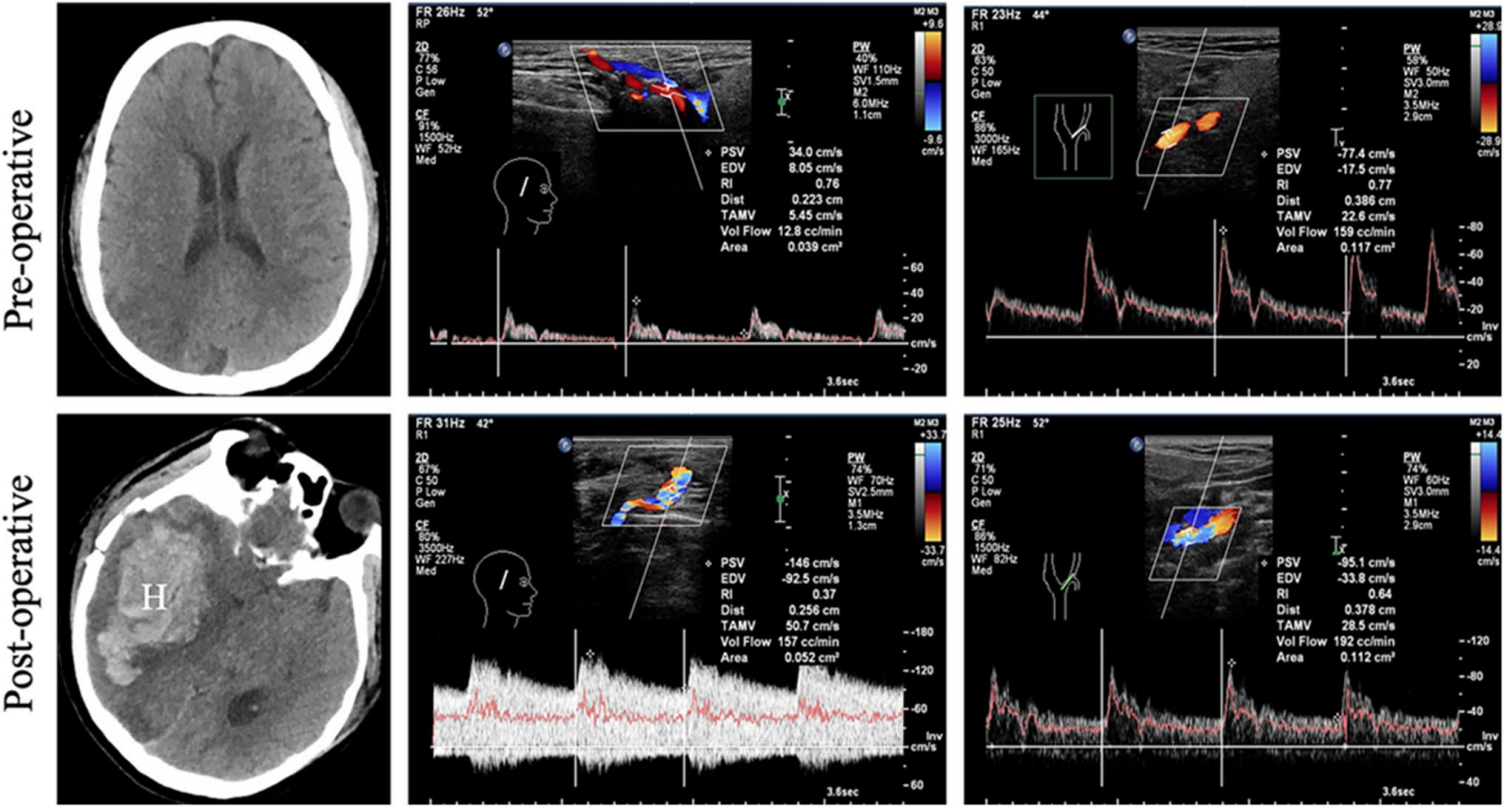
A 44-year-old male MMD patient underwent right-side STA-MCA bypass surgery. One week after, the patient was found CHS manifesting with headache, vomiting, and consciousness disturbance. Emergency CT showed a large hematoma in the right frontotemporal insular lobe, complicated with basal ganglia hemorrhage and cerebral hernia (the left column). On TCCS, PSV at the proximal end of STA increased sharply more than 4 folds, RI decreased by half (the middle column), and the flow velocity of ECA slightly increased (the right column). H indicates hematoma; CHS, cerebral hyper-perfusion syndrome. Image courtesy of Dr. Chenyun Zhou, Department of Ultrasound, West China Hospital
